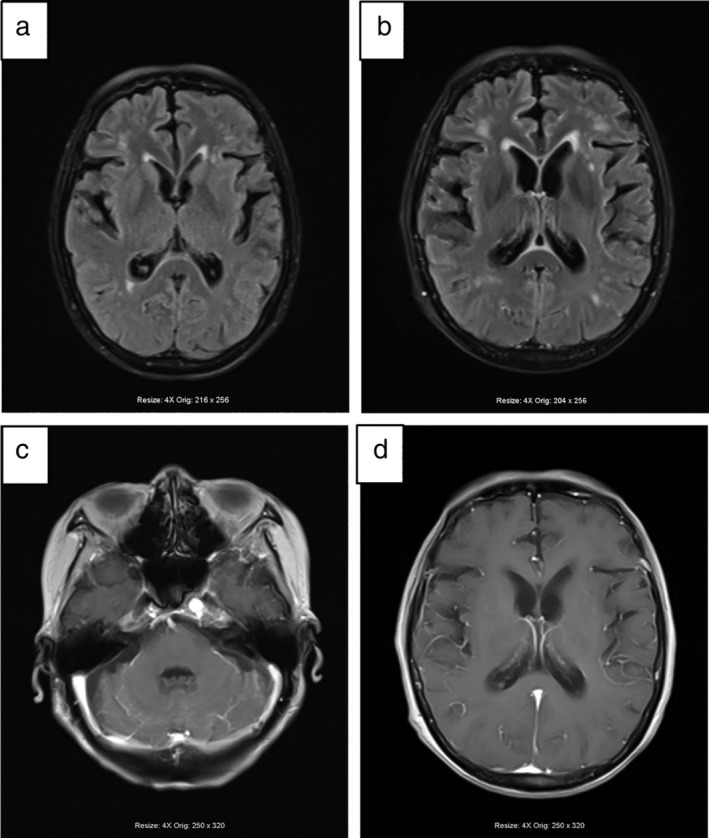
Brain MRI scan demonstrated metastatic meningeal carcinomatosis. (a) Axial fluid‐attenuated inversion recovery (FLAIR) image showed slightly hyperintense in the bilateral temporal‐parietal lobe sulcus. (b) Axial view of enhanced FLAIR: after intravenous injection of a contrast agent, abnormal linear enhancement changes were observed in the bilateral frontotemporal‐occipital sulcus. (c) Enhanced axial view of T1WI: abnormal linear contrast‐enhanced changes were observed in the ventral and dorsal medulla oblongata, bilateral cerebellar hemisphere plicate meninges. (d) Enhanced axial view of T1WI: abnormal linear contrast‐enhanced changes in the duodenal meninges in bilateral cerebral hemispheres were seen.
